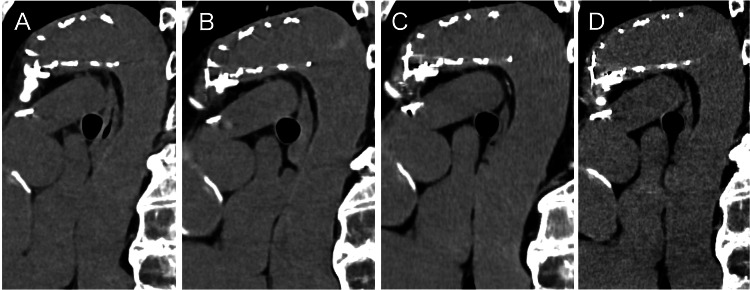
Downstream aorta after operation The computed tomography scan of the downstream aorta at (A) six months after operation, (B) two years after operation, (C) four years after operation, and (D) six years after operation.
